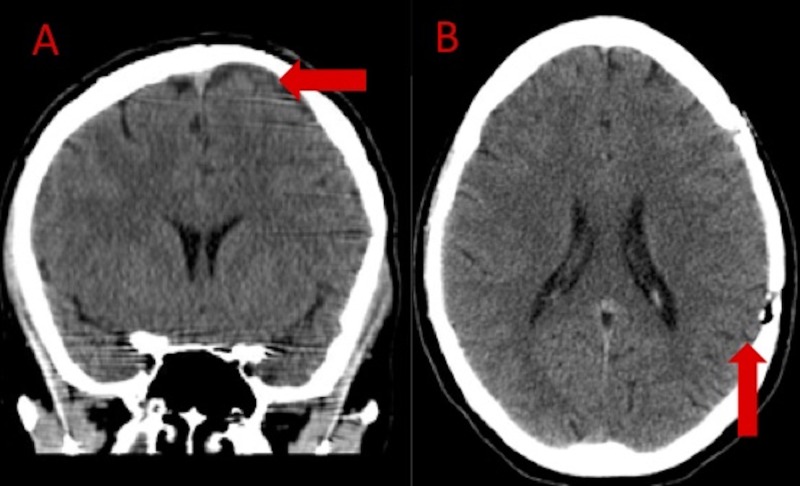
Noncontrast head computed tomography two months after craniotomy A: Coronal view demonstrating resolution of subdural hematoma as well as no residual arachnoid cyst (arrow). B: Axial head computed tomography demonstrating resolution of left cerebral convexity subdural hematoma (arrow).
